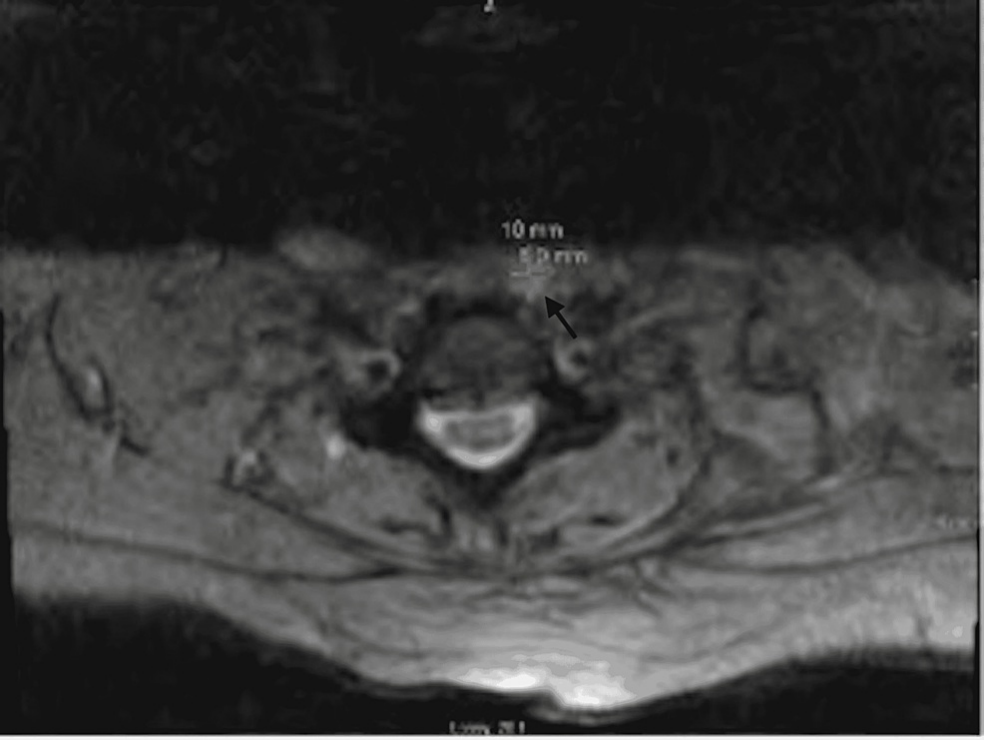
MRI Cervical Spine MRI image showing abnormal signal with prevertebral edema and fluid collection concerning for vertebral discitis-osteomyelitis with small prevertebral abscess, marked with an arrow.
